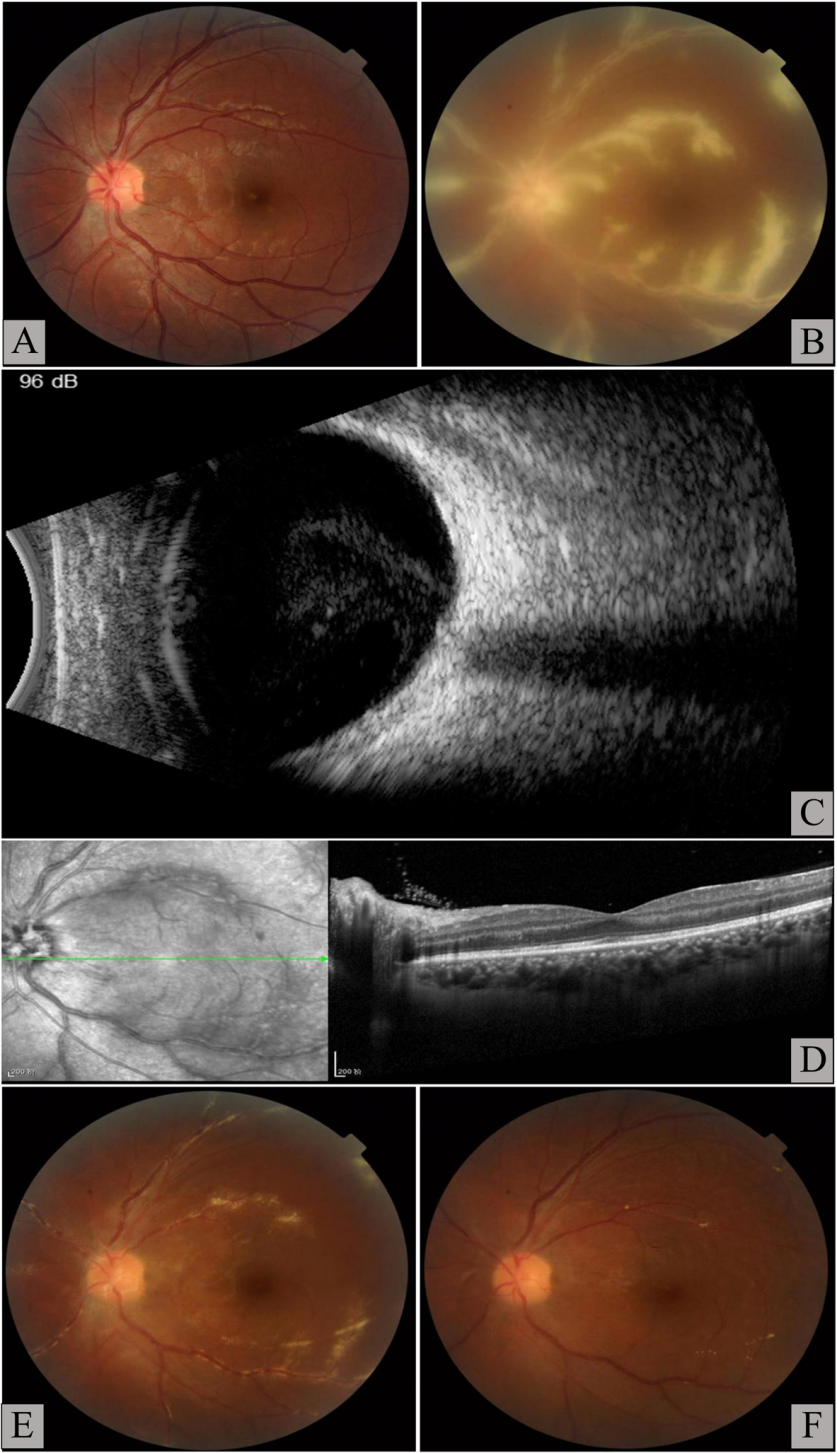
Changes observed in the patient’s eye examination. A Normal bilateral eyes appearance before antiretroviral therapy (ART). B After 5 weeks of ART, the left eye showed sheathing of the retinal vessels appearing like frosted branches of a tree without haemorrhages, necrosis, and occlusion. C Eye ultrasound revealed mild vitreous haze in the left eye after 5 weeks of ART. D No oedema in the macular area by optical coherence tomography were seen after 5 weeks of ART. E After 6 weeks of anti-CMV treatment, frosted branch angiitis in the left eye had significantly improved. F After 6 months of anti-CMV treatment, the retinal perivenous exudate in the left eye had resolved
